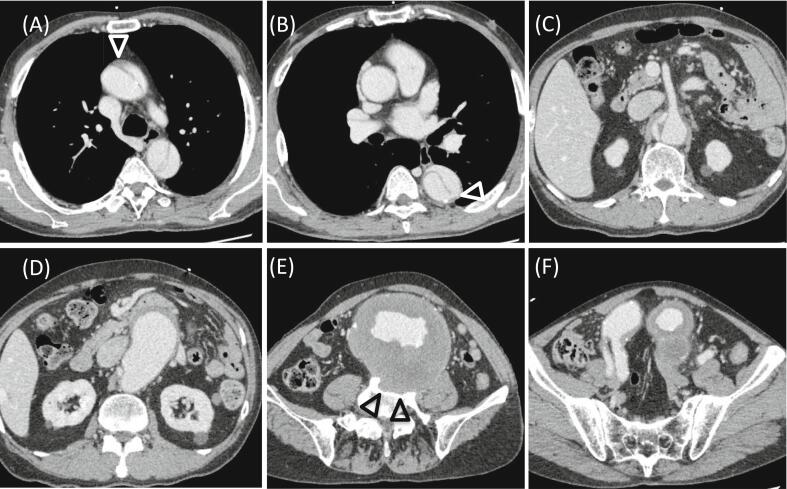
Computed tomography (CT) images on initial admission. (A) The aortic dissection extends from the ascending aorta, and the false lumen is patent. Thrombosis is observed in a small portion of the false lumen of the ascending aorta (arrowhead). (B) The main entry is at the descending aorta (arrowhead). (C) The aortic dissection is noted at the level of the superior mesenteric artery and extends to the vicinity of the right renal artery. (D) The abdominal aortic aneurysm (AAA) has a huge reverse tapering neck. (E) The AAA measures 11.5 × 9.8 cm. Part of the vertebral body is destroyed (arrowheads). (F) The aneurysm extends to the common iliac arteries bilaterally.
